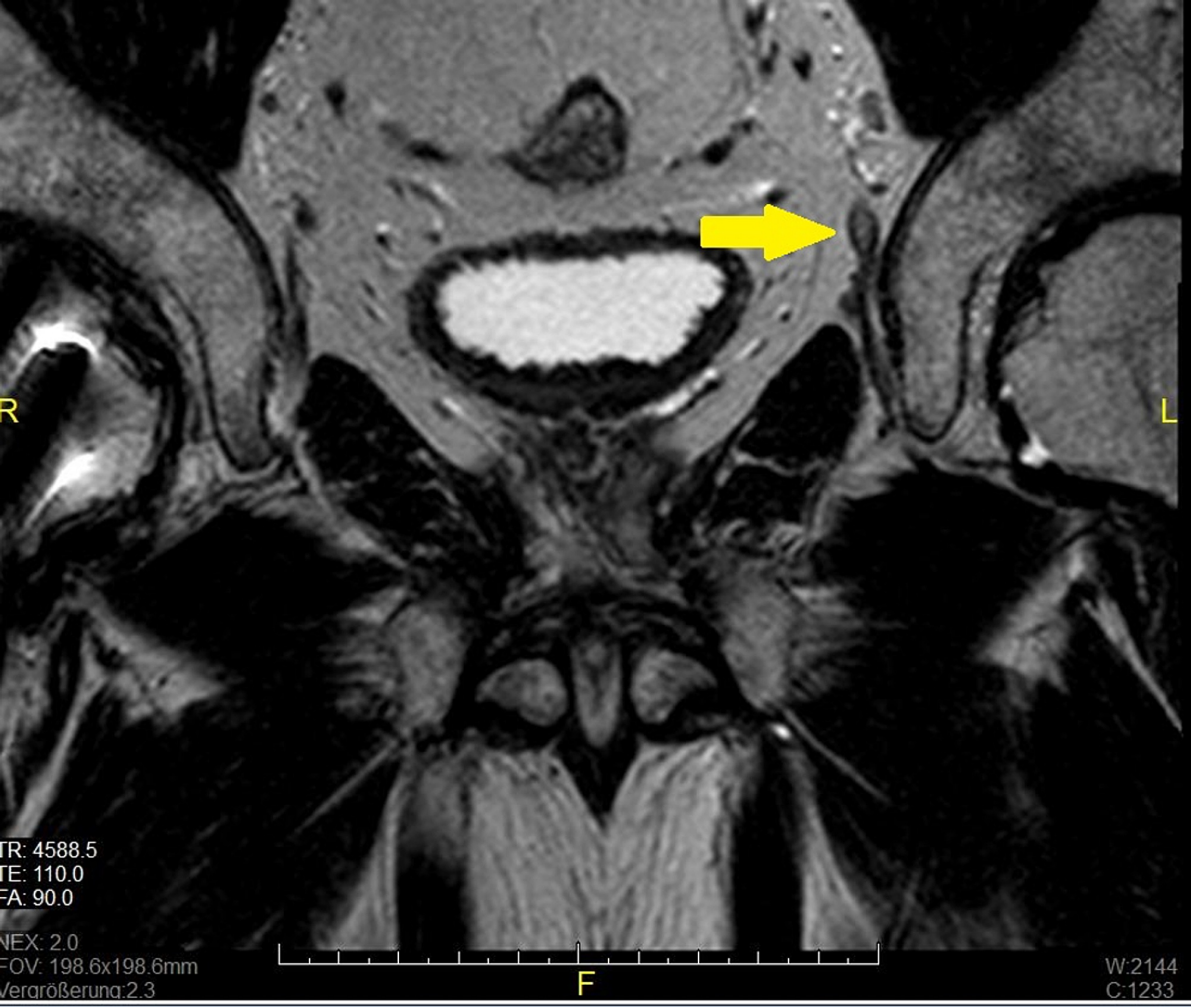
Preoperative MRI of the prostate and the pelvis The coronal sequence of the preoperative multiparametric MRI of the prostate and the pelvic area. Thickening of the neuraxon in the pars pelvica of the left obturator nerve (arrow) MRI: magnetic resonance imaging
